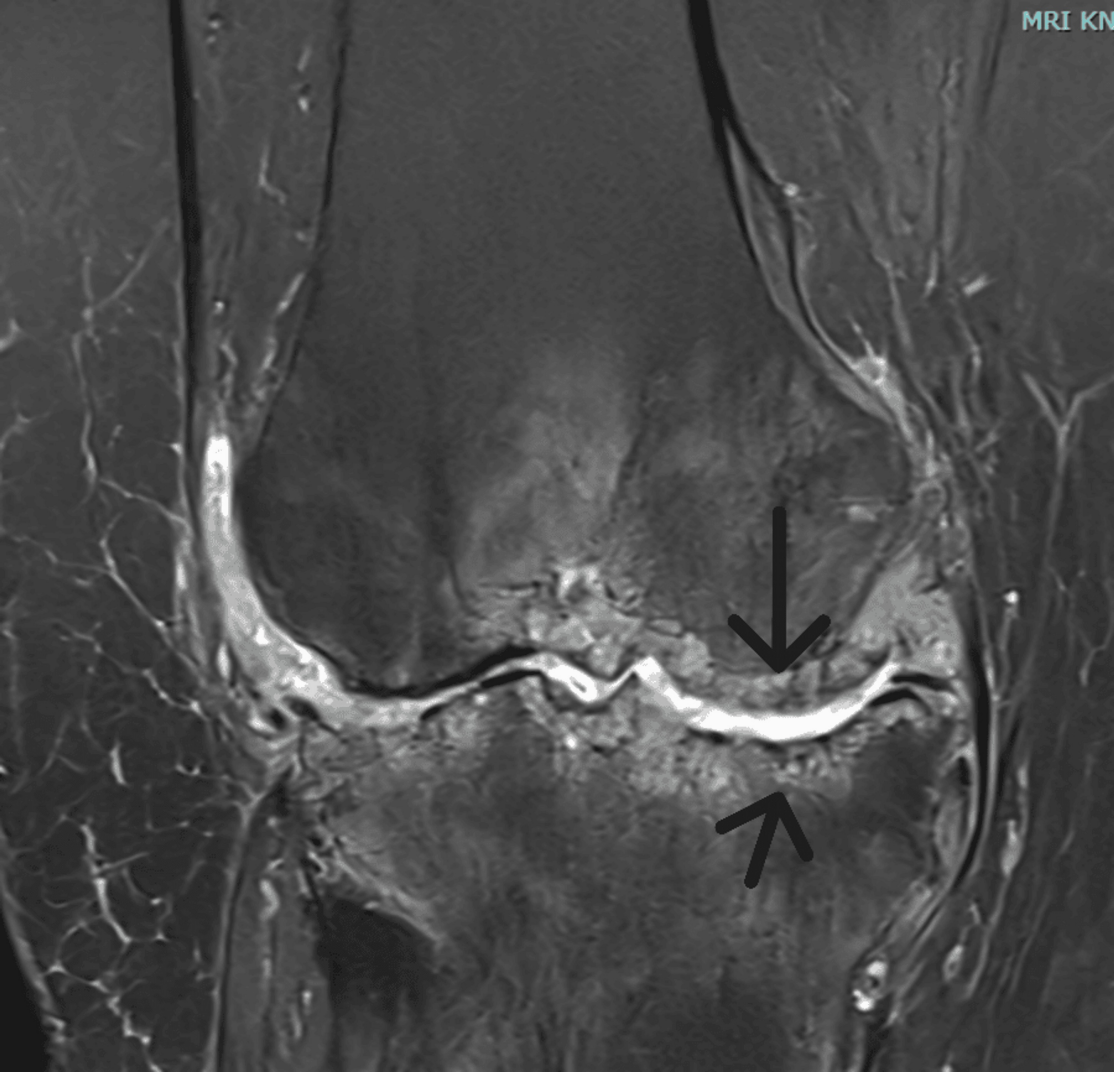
Τ2 weighted image, MRI of the right knee, coronal view. Arrows pointing towards evidence of joint line erosion.
